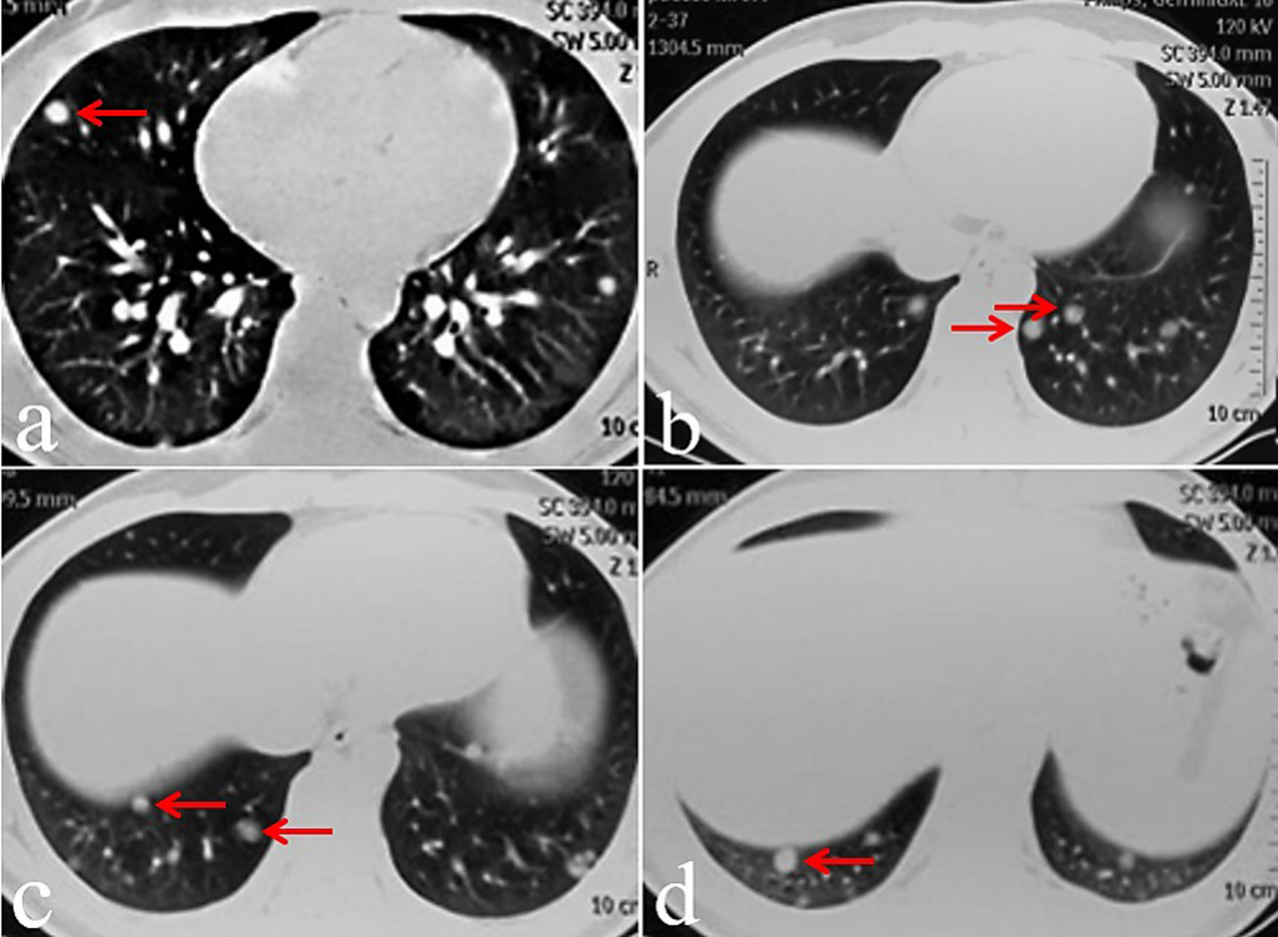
A March 2015 chest CT revealed multiple pulmonary nodules likely representing metastatic disease
